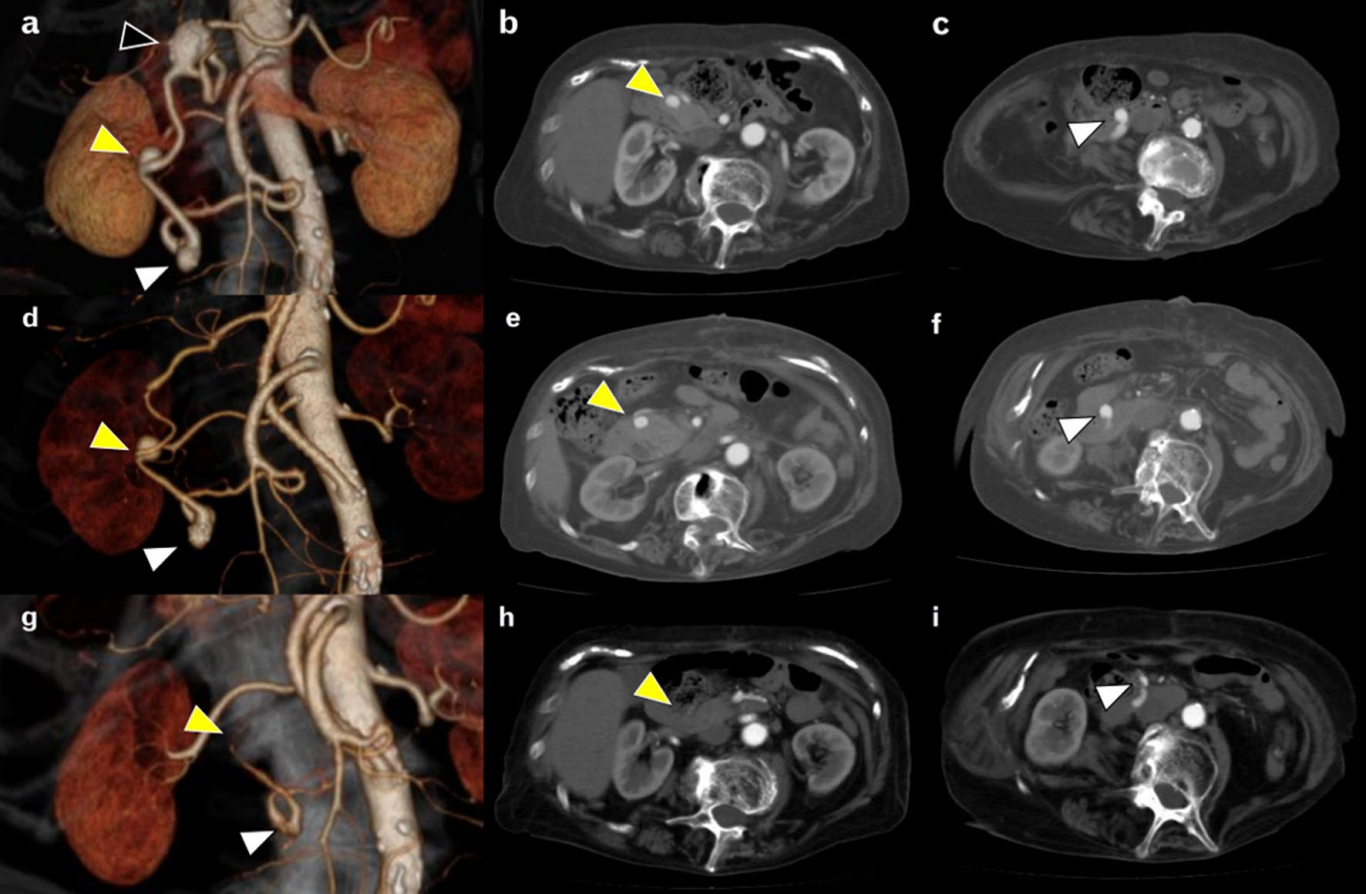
Three-dimensional computed tomography and contrast-enhanced computed tomography. a Three-dimensional computed tomography (3D-CT) before bypass surgery revealed a 20 × 17 mm superior pancreaticoduodenal artery aneurysm (SPDAA) (black arrowhead), 10 × 10 mm inferior pancreaticoduodenal artery aneurysm (IPDAA) (yellow arrowhead), 11 × 11 mm IPDAA (white arrowhead), and dilated pancreaticoduodenal arcade (PDA). b 10 × 10 mm inferior pancreaticoduodenal artery aneurysm (yellow arrowhead). c 11 × 11 mm inferior pancreaticoduodenal artery aneurysm (white arrowhead). d–f 3D-CT and CT 1 month after bypass surgery and aneurysmectomy revealed regression of the IPDAAs and a decrease in the dilated PDA arcade. g–i One year after surgery revealed complete regression of IPDAAs with progression of thrombosis and a decrease in blood flow. Blood flow and aneurysms were mostly diminished
